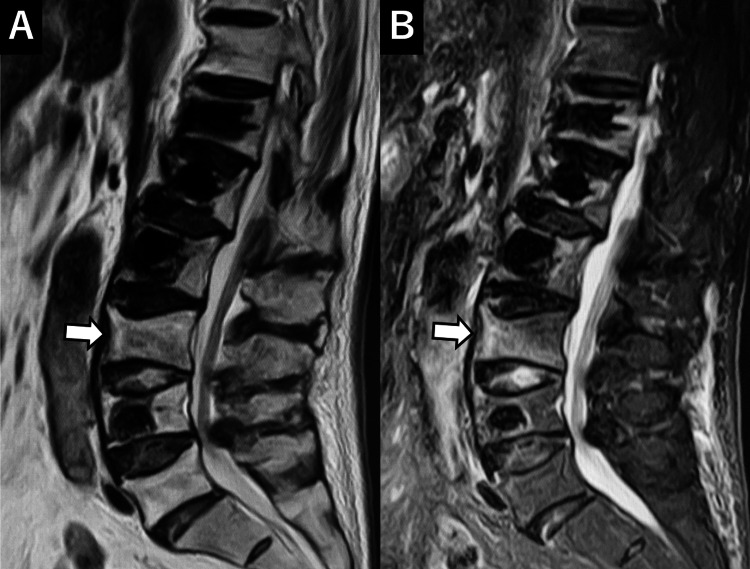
Pre-third surgery MRI A: T2-weighted image (T2WI), B: Short T1 inversion recovery (STIR). A new vertebral compression fracture is seen at L3 (arrow).
